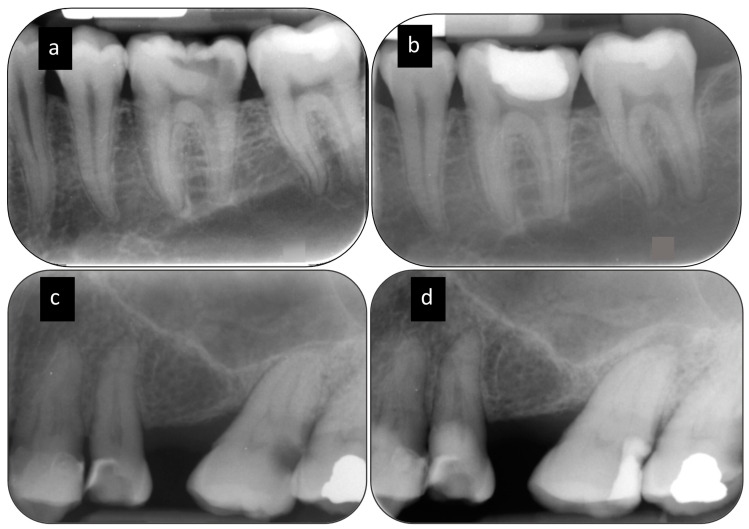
Periapical radiolucency detected by PA radiograph. (a) Periapical radiograph of the lower left first molar with a healthy PDL space at T0 (b) T24 PA radiograph shows healthy periapical tissue. (c) Periapical radiograph of the upper left second molar with a healthy PDL space at T0 (d) T24 PA radiograph shows at the periapex of the tooth a periapical radiolucency.
